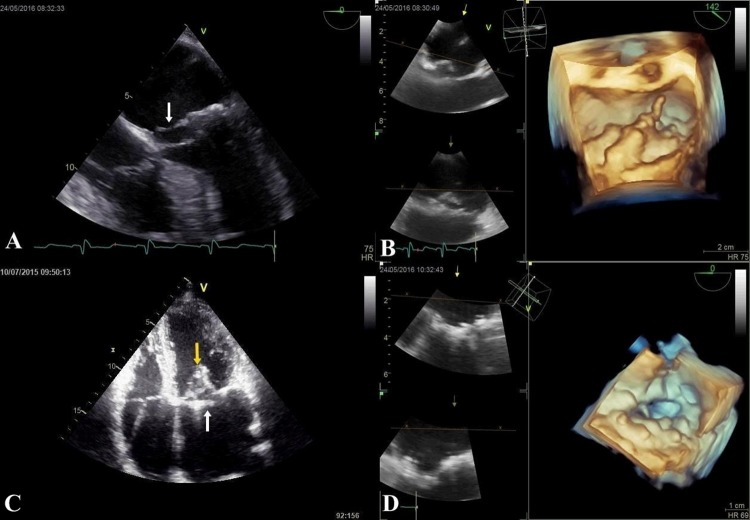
2D and 3D assessment of patient with MVP A. 2DTEE pre-operative imaging showing flail posterior leaflet (arrow); B. 3DTEE pre-operative imaging showing flail posterior leaflet( P2); C. 2D TTE apical four chamber view - post-operative imaging showing perfect coaptation (yellow arrow) and lack of leaflet prolapse (white arrow); D. 3DTEE post-operative imaging showing perfect coaptation and lack of leaflet prolapse.
